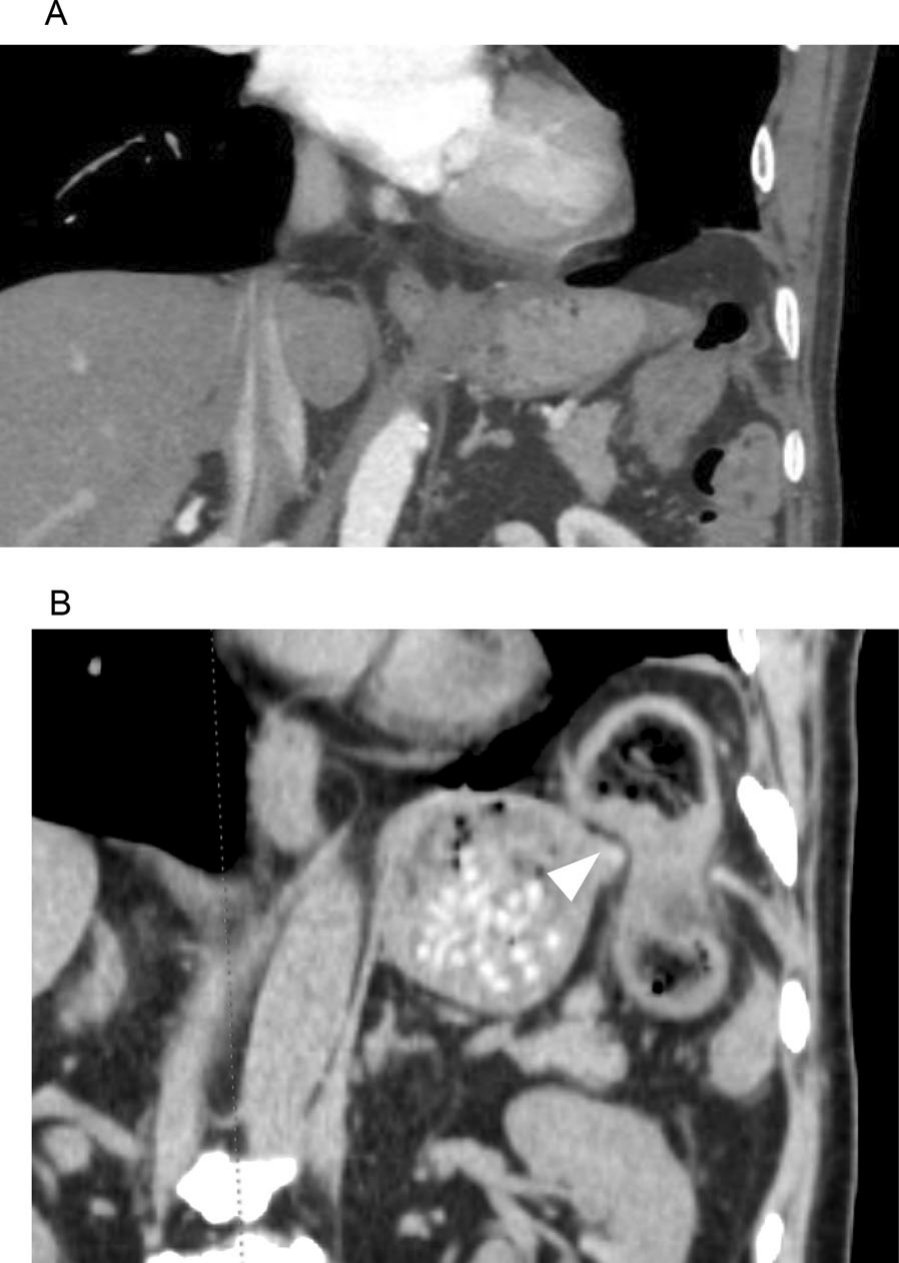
Preoperative CT images. CT images show intra-abdominal tissue evacuated into the thoracic cavity in the absence of pain (A) and presence of pain (B). We confirmed the colon incarceration (white arrowhead)
